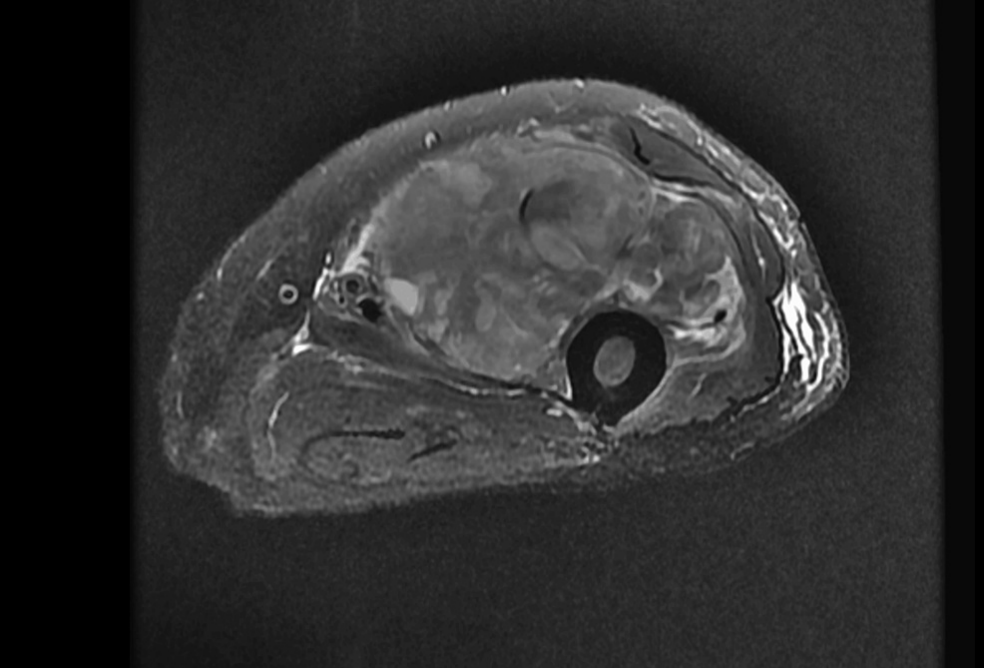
Magnetic resonance imaging showing a 12.2 cm mass deep in the sartorius muscle close to the rectus femoris.
